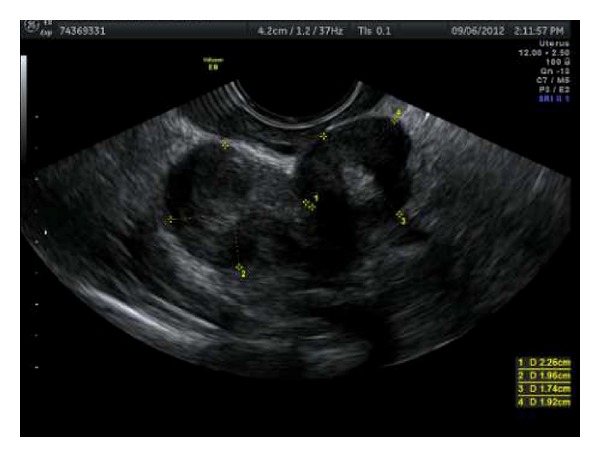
Ultrasound: multiple solid masses in the pouch of Douglas (23 mm × 20 mm and 17 mm × 19 mm), with myometrial aspect and central echogenic parts. They are attached to the left ovary and rectosigmoid but without invasion of its muscular wall.
